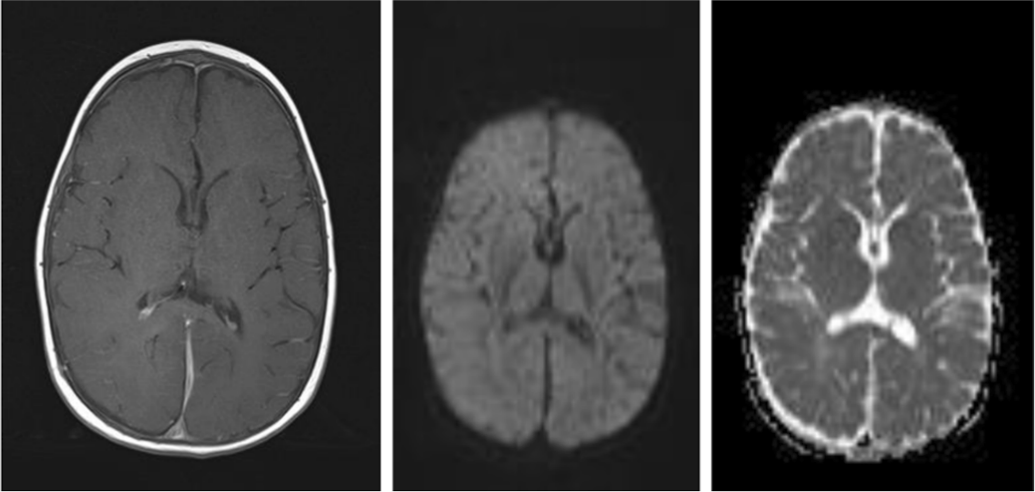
Magnetic Resonance Imaging 12 weeks after admission.
